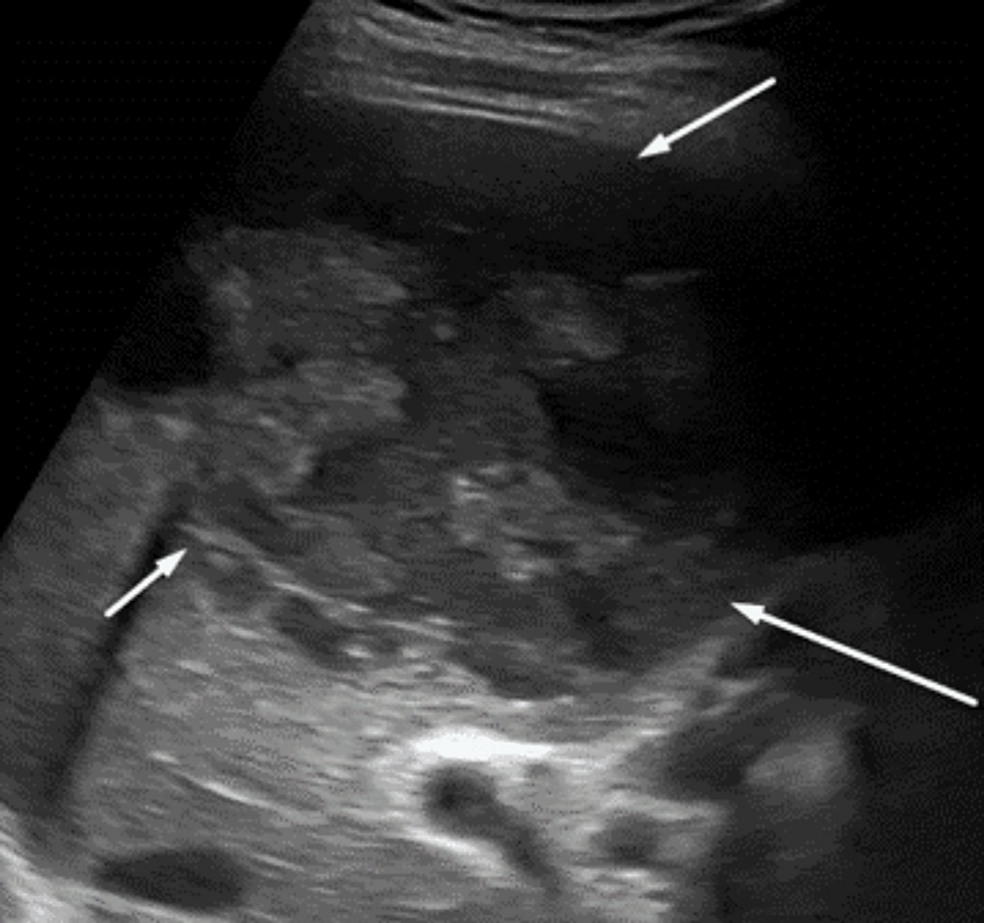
Ultrasound of the abdomen showing liver abscess at admission.
